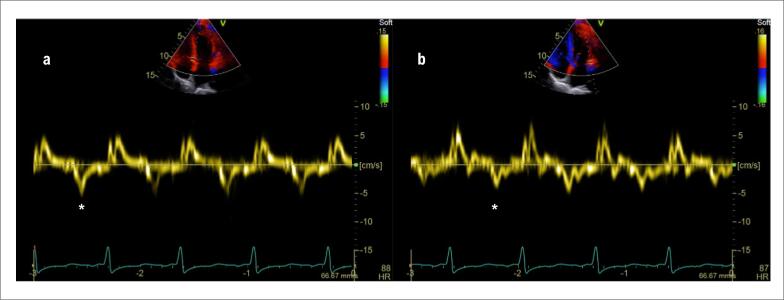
Imagens de Doppler tecidual em anel mitral. Há redução da velocidade da onda e’(*) tanto no Doppler tecidual do anel mitral medial (a) quanto no do lateral (b). Ambas as velocidades se encontram abaixo de 4 cm/s (VR > 8 cm/s). (Imagens de arquivo pessoal dos autores).
